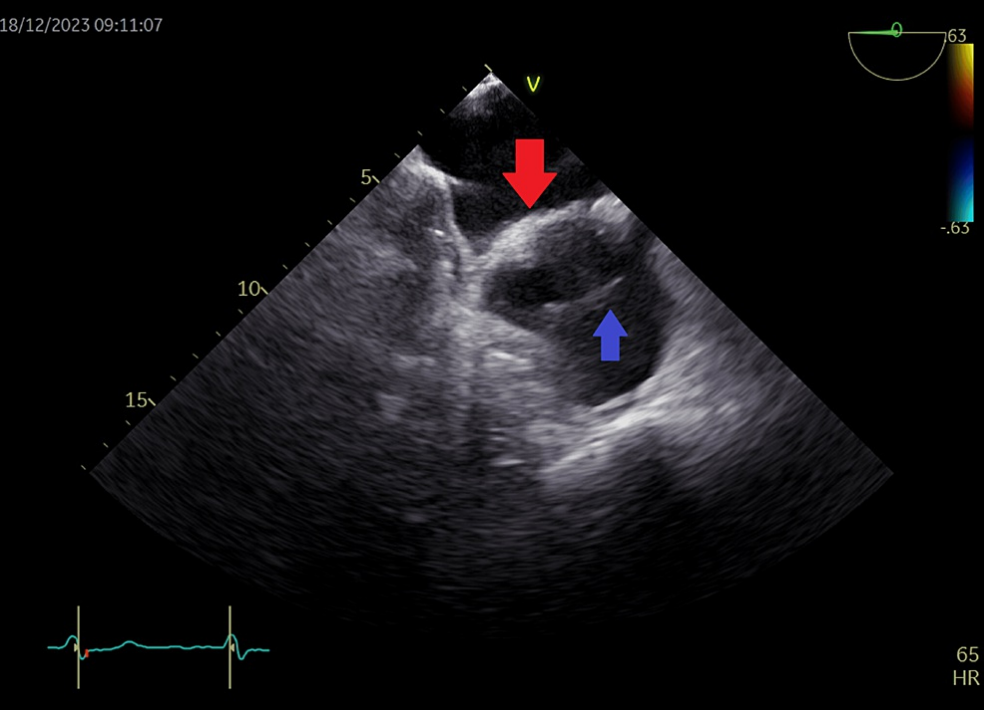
Transesophageal echocardiography view of the left atrium (red arrow) and right atrium (blue arrow) also showing the cor triatriatum dexter membrane dividing the right atrium (blue arrow).
